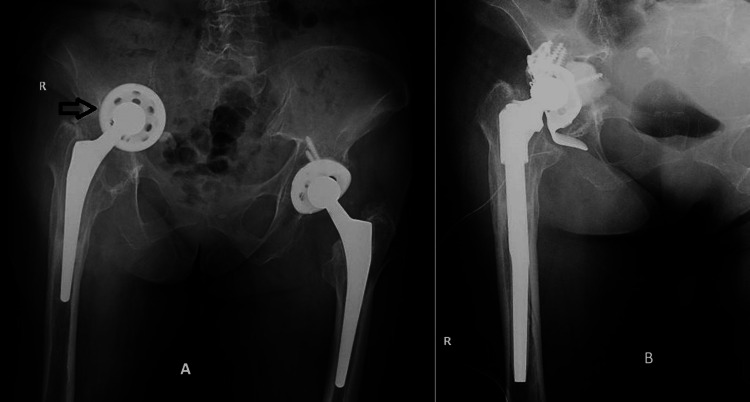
A. Preoperative antero-posterior pelvic X-ray. B. Postoperative right hip X-ray.
